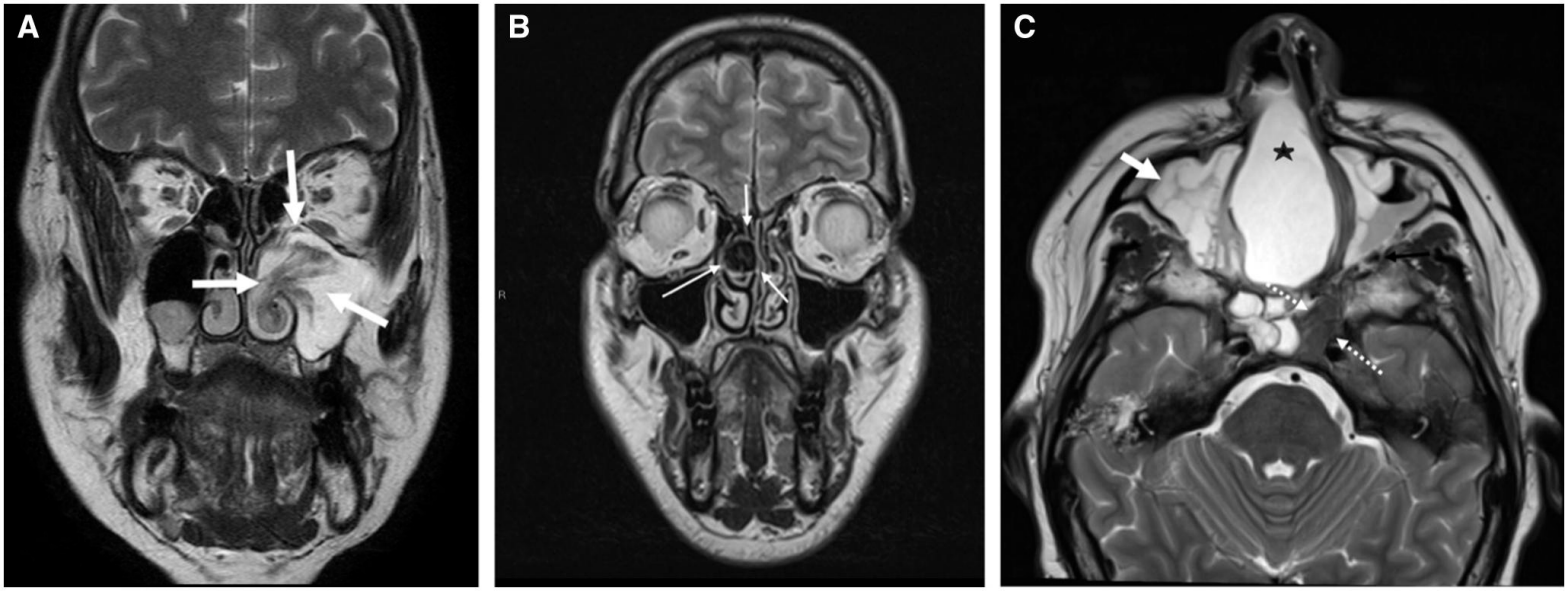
Benign sinonasal pathology. (A) Left nasoantral inverted papilloma: coronal T2-weighted MR image showing the classic cerebriform morphology (white arrows) reflecting its pattern of growth deep to an intact respiratory basement membrane and oedema of the adjacent stroma (type 3 Schneiderian polyp). The lesion insinuates into the maxillary antrum via the native ostium. The posterior accessory ostium can also be a route of IP extension. (B) Fungal ball within a right middle turbinate concha bullosa: coronal T2-weighted MRI showing the classic signal drop-out (thin white arrow) seen with fungal tissue owing to paramagnetic hyphal contents. This will typically show increased (albeit variable) signal on the accompanying T1-weighted sequence; therefore, scrutiny of all sequences is key to avoid confusing this with aerated air cells. There is no erosion of the nasal osteo-cartilaginous structures, but mucocoeles can develop due to obstructing fungal balls. (C) Mega polyp within the nasal cavity: axial T2-weighted MRI shows a large mega polyp filling and expanding the right nasal cavity (star) obstructing the ostiomeatal units. The polyp demonstrates high T2-weighted signal. There is mucosal thickening and submucosal oedema within the maxillary antra (short white arrow) and right sphenoid chamber, and low-signal fungal tissue within the obstructed left sphenoid chamber (dotted white arrow).
